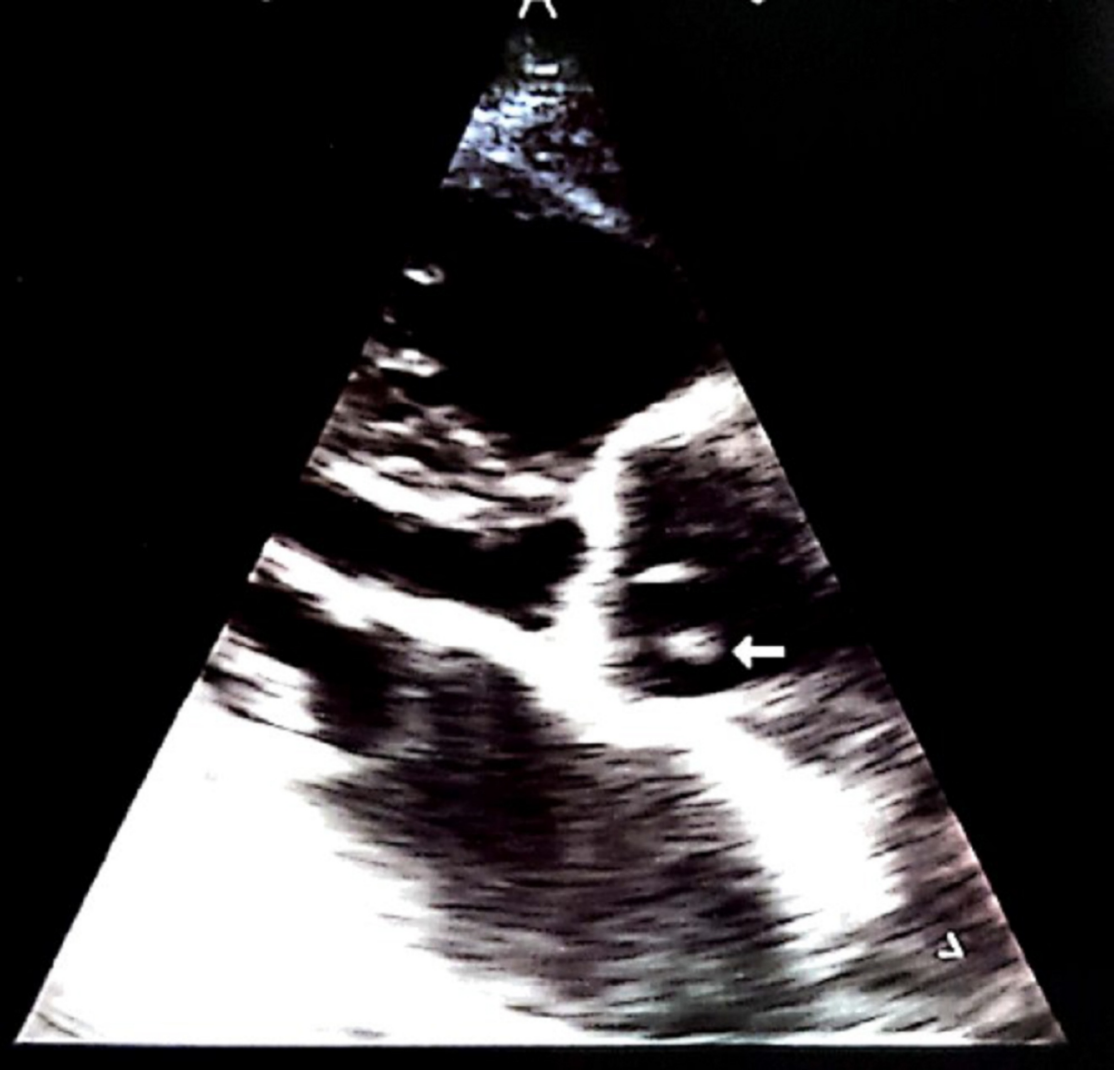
Patient's echocardiogram depicting valvular vegetations Mobile vegetations on the non-coronary cusp of the aortic valve can be seen (white arrow).
